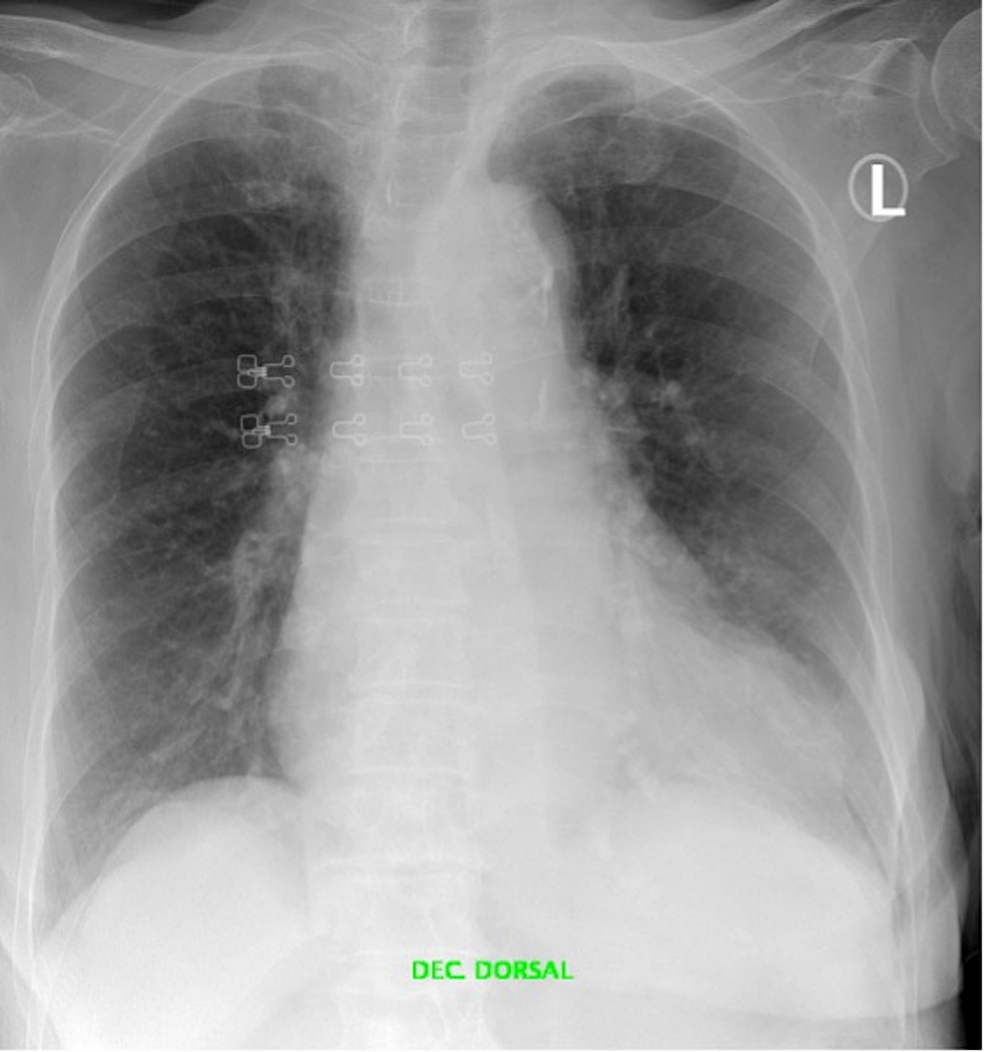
Chest X-ray with mediastinal widening and an enlarged cardiomediastinal silhouette
